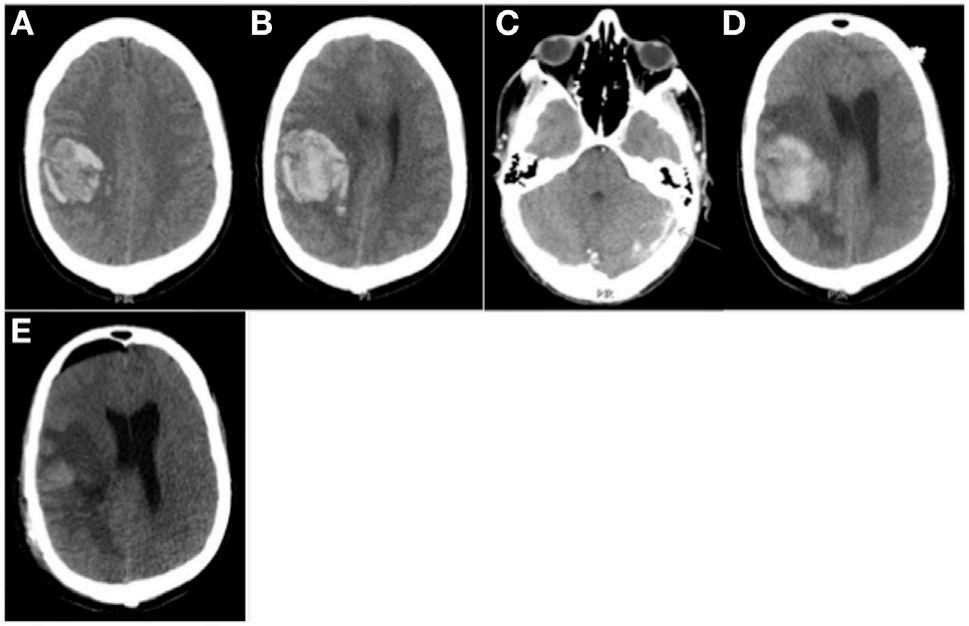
Patient 1. CT head (A,B,D) and CT venography (C) prior to surgery: (A) Day 6: large right frontal cerebral hematoma (40 mL), moderate mass effect with 4 mm of maximal brain midline shift. (B) Day 7: hematoma volume increase up to 45 mL. (C) Day 13: left lateral venous sinus thrombosis. (D) Day 14, just before surgery: increased mass effect (10 mm brain midline shift) with peripheral edema. CT head after surgery: (E) Day 16: decreased mass effect (6 mm brain midline shift).
